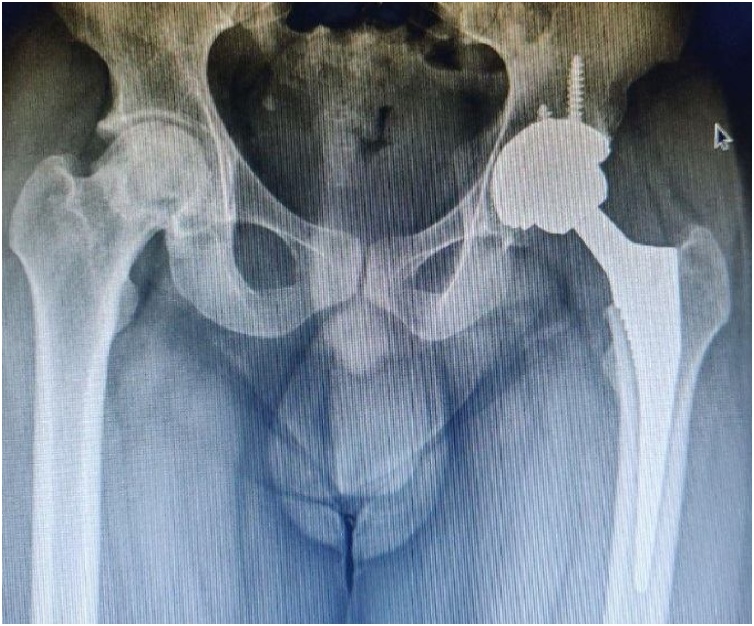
Showing postoperative AP radiograph a normal seated acetabular component and liner.
